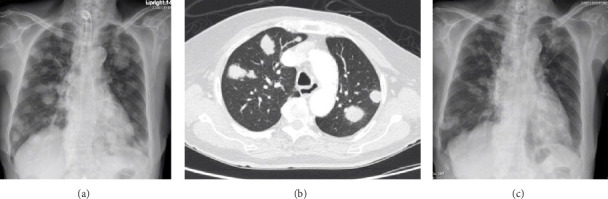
Chest imaging. (A) Chest X-ray: multiple pulmonary nodules of varying sizes in both lungs; (B) CT chest: multiple pulmonary nodules and subpleural masses/nodules in both lungs, ranging from 0.3 to 3 cm in size; and (C) chest X-ray after treatment with pazopanib: decreasing in size of all multiple pulmonary nodules and masses.
